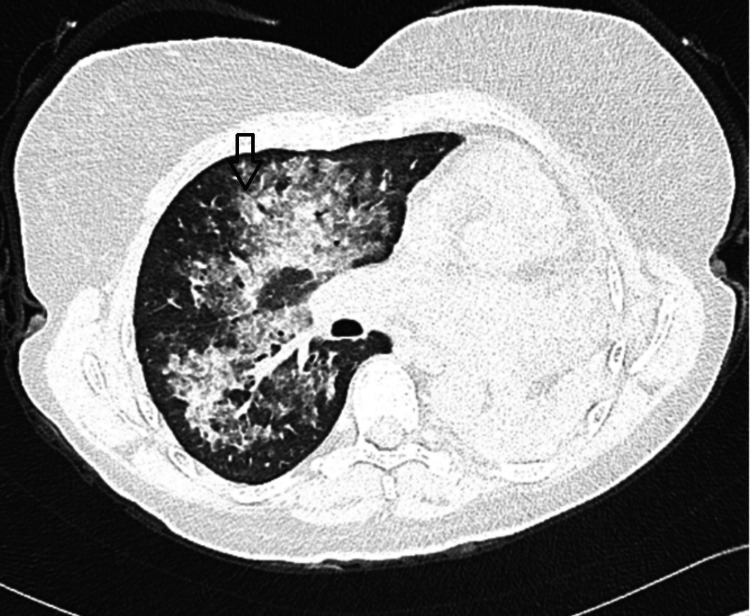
High-resolution computed tomography demonstrating right-sided ground-glass opacities (arrow) with subpleural sparing suggestive of DAH, a severe GPA complication with long standing total left lung collapse due to GPA-induced left main stem bronchus narrowing. Ground-glass opacities (i.e., “hazy areas reflecting alveolar filling”). DAH – diffuse alveolar hemorrhage, GPA – granulomatosis with polyangiitis
